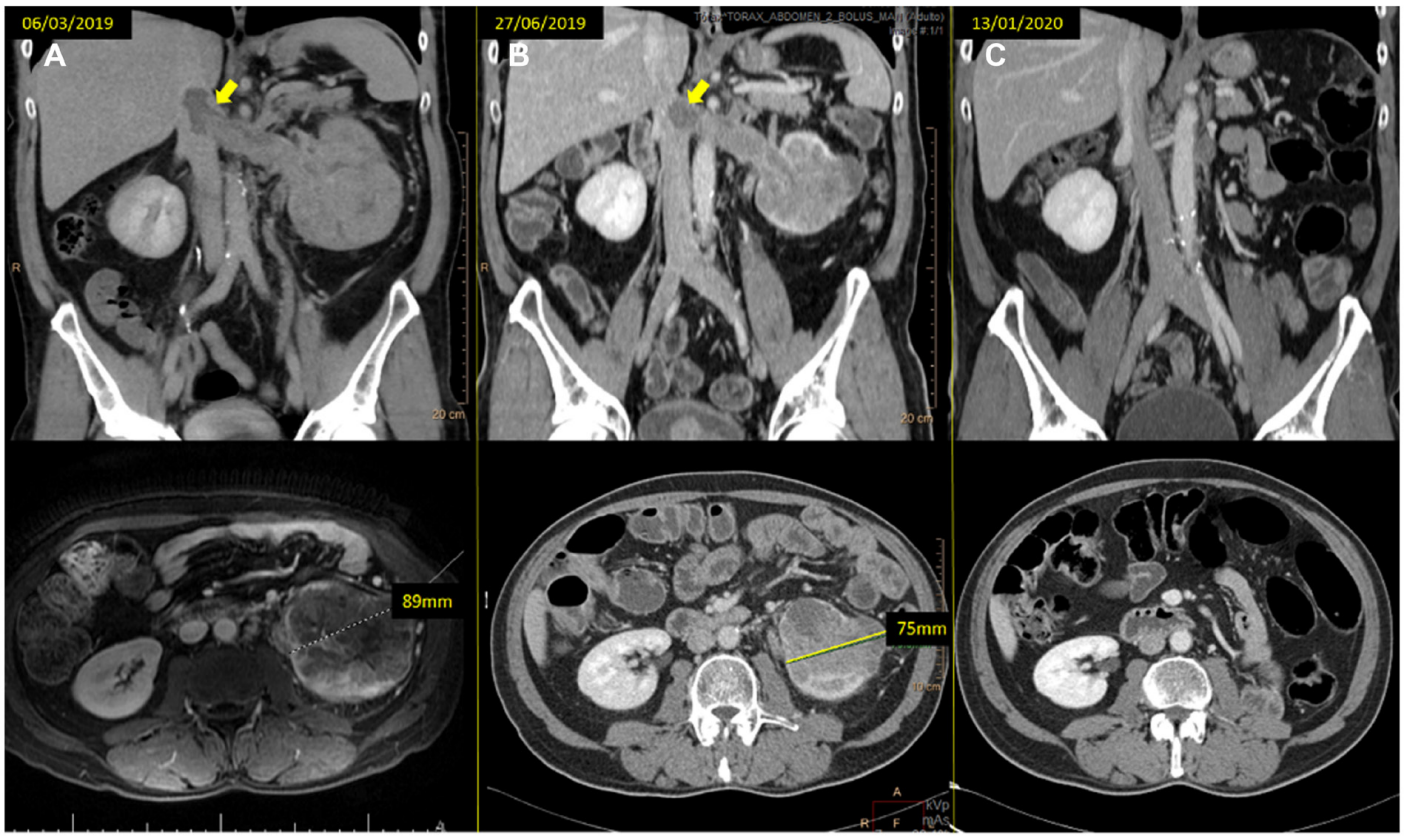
(A) baseline CT scan and (B) the reassessment CT scan at 12 weeks of treatment, showing the tumor thrombus downsizing (arrows) and the left kidney mass response (from 89 to 75 mm). (C) last reassessment CT scan where the patient remains progression free.
